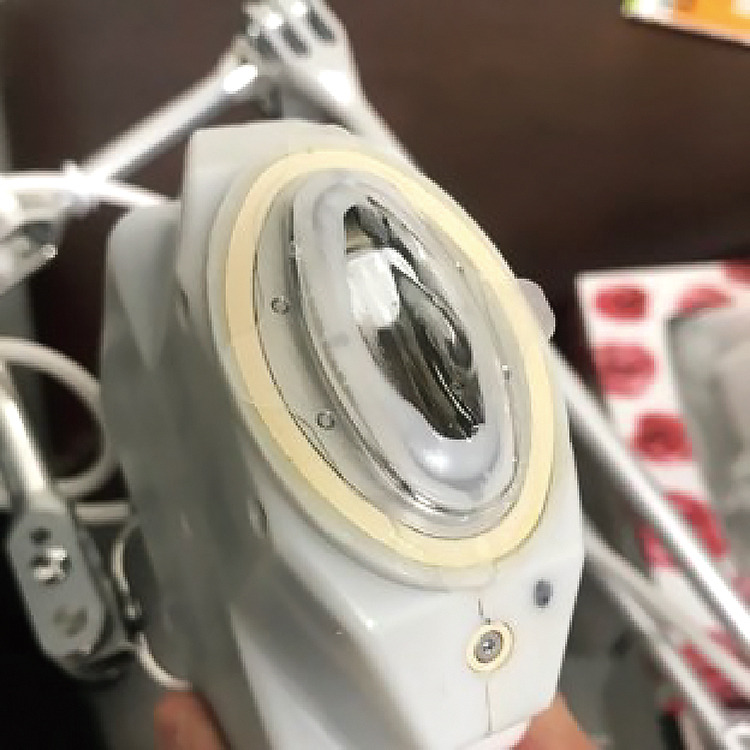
Probe photographs obtained using a wide-angle ultrasound diagnostic device A cap filled with a physiological saline solution was fitted and the tip of the contained probe was free to move to trace an arc (Extracted from Figure 1 from Sekido K, Murayama K, Mizuguchi T, Sakurai R, Tanikawa A, Horiguchi M. Eyeball in the normal eye evaluated using a wide-angle ultrasound diagnostic device. Folia Japonica de Ophthalmologica Clinica. 2020;13:717–21 with permission)
